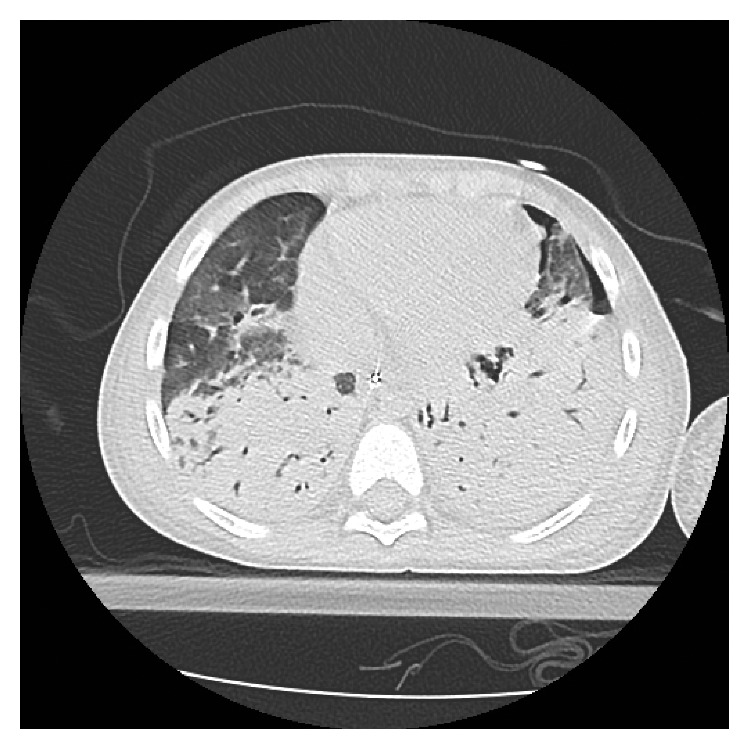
Computed tomography image revealing dense consolidation and pneumothorax. The clinical findings were in keeping with bronchopleural fistula.
